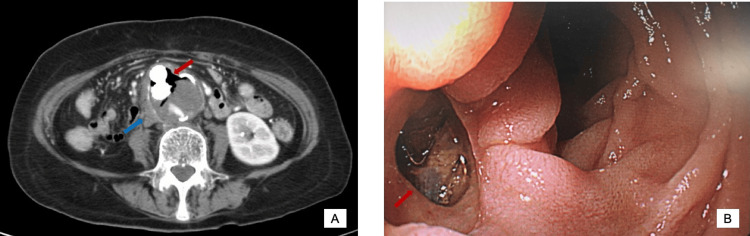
Contrast-enhanced CT of the abdomen (A) and upper gastrointestinal endoscopy (B) A: The image reveals air within the aneurysm (red arrow) and thickening of the aortic wall (blue arrow). B: The image of upper gastrointestinal endoscopy in the descending duodenum indicates an aortoduodenal fistula and the stent visible through the fistula (red arrow) CT: computed tomography
